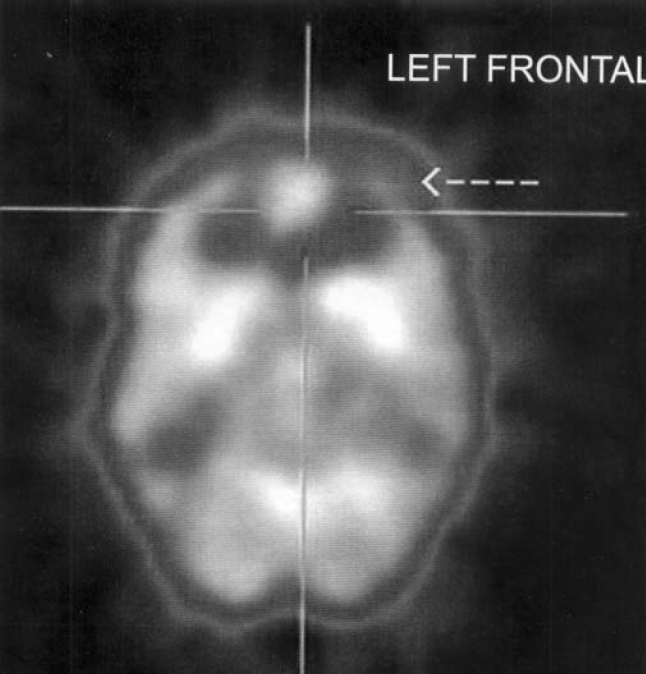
SPECT scan showing reduced perfusion in left frontal lobe in subject no. 5
